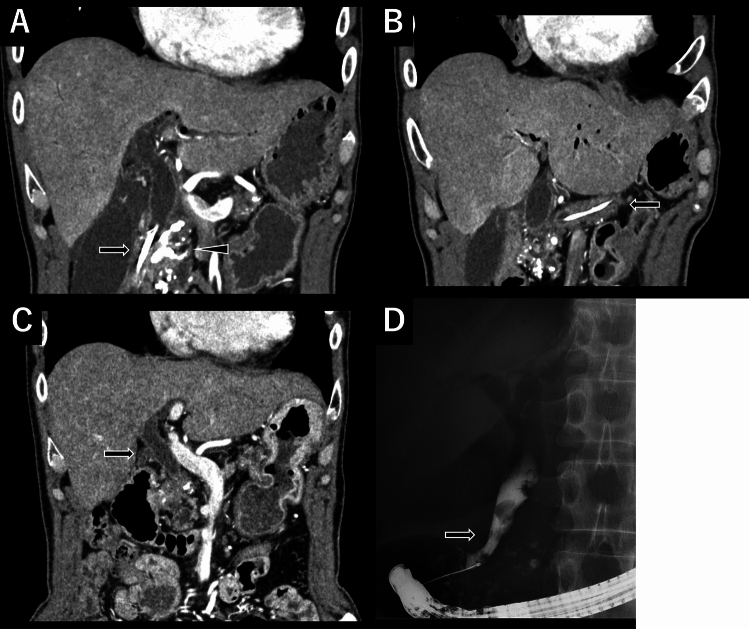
Imaging studies of a case with improvement of a CBD stricture 53 months after the Frey procedure. A Preoperative computed tomography (CT) revealing calcification in the pancreatic head (arrowhead) and a CBD stent in place (arrow). B Preoperative CT imaging showed the pancreatic duct stent in place. C At 53 months after surgery, the CBD stricture had resolved. D Although CBD dilation was still observed, the CBD stent was no longer required
